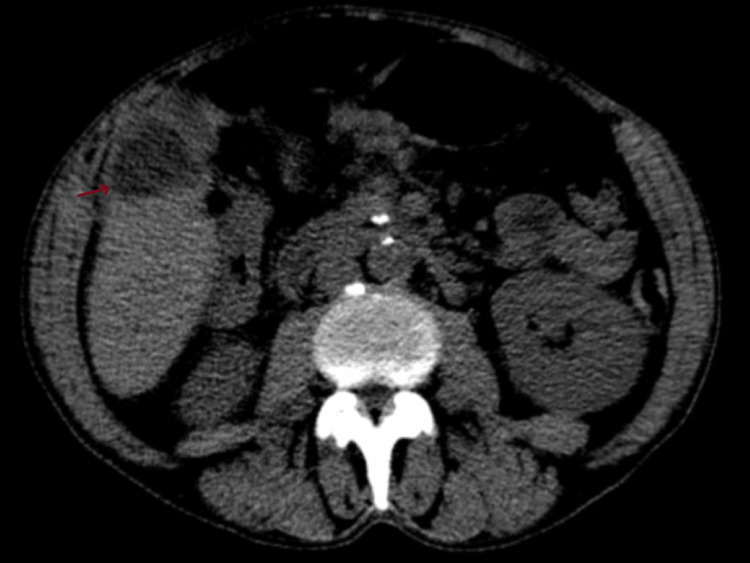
Abdominal CT scan showing nodular hepatic lesions (arrow).
